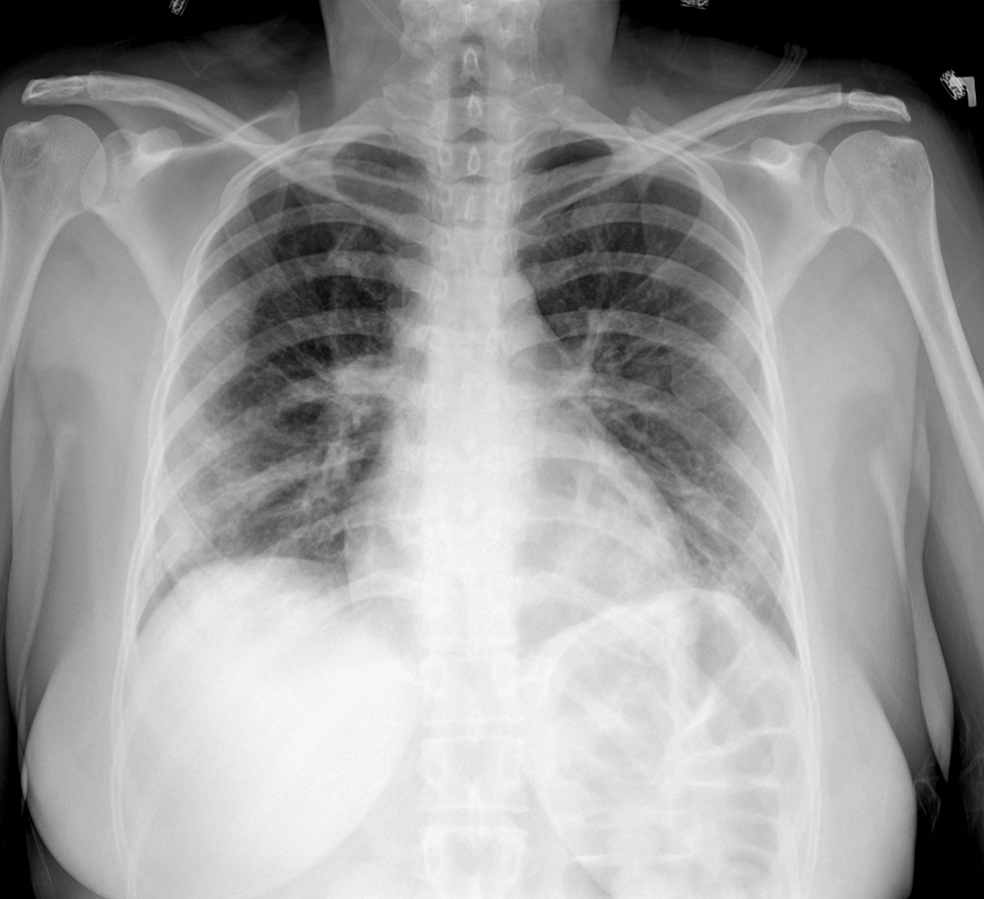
Chest x-ray of a 44-year-old female with COVID-19 viral pneumonia
